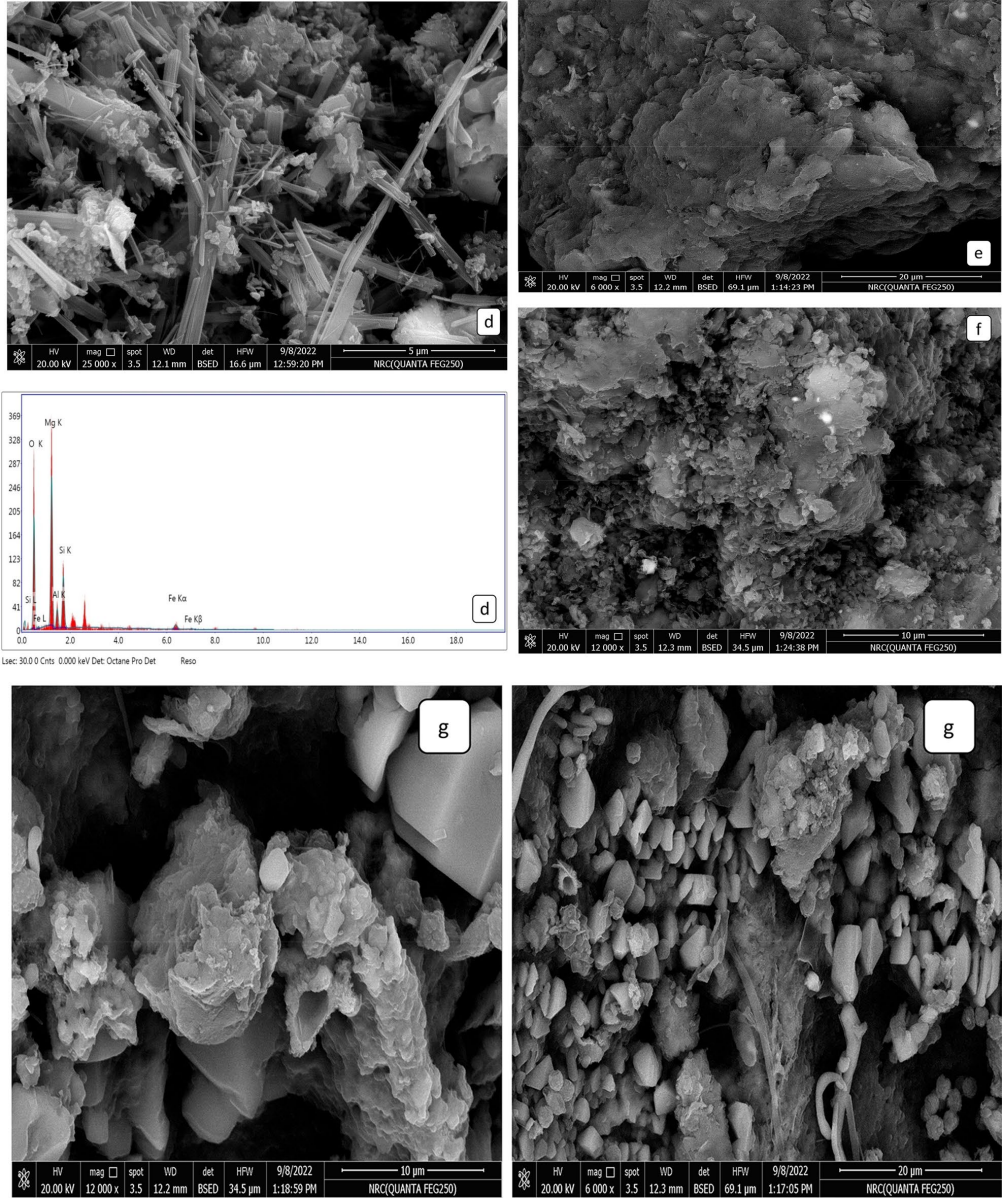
TEM (a) nano bentonite, (b) MgO impregnated clay, and SEM images and Energy dispersive X-ray analysis (c) nano bentonite within EDX, (d) MgO impregnated clay within EDX (e) nano bentonite after adsorption MG, (f) MgO impregnated clay after adsorption MG and (g) after adsorption MG by fungi low and high magnification respectively.
